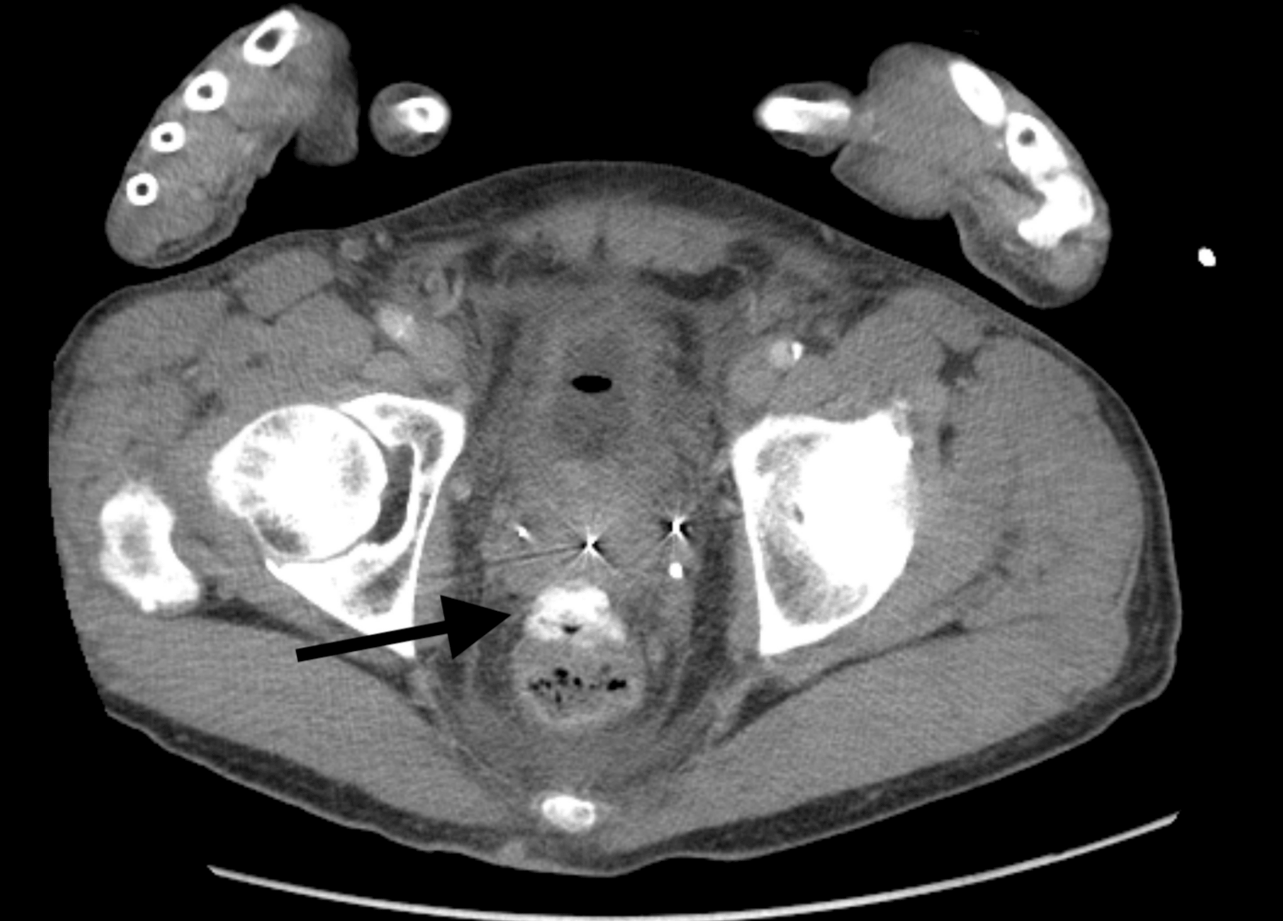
Axial CT image at the level of the prostate demonstrating a fluid collection with features suggestive of a possible abscess (black arrow).
